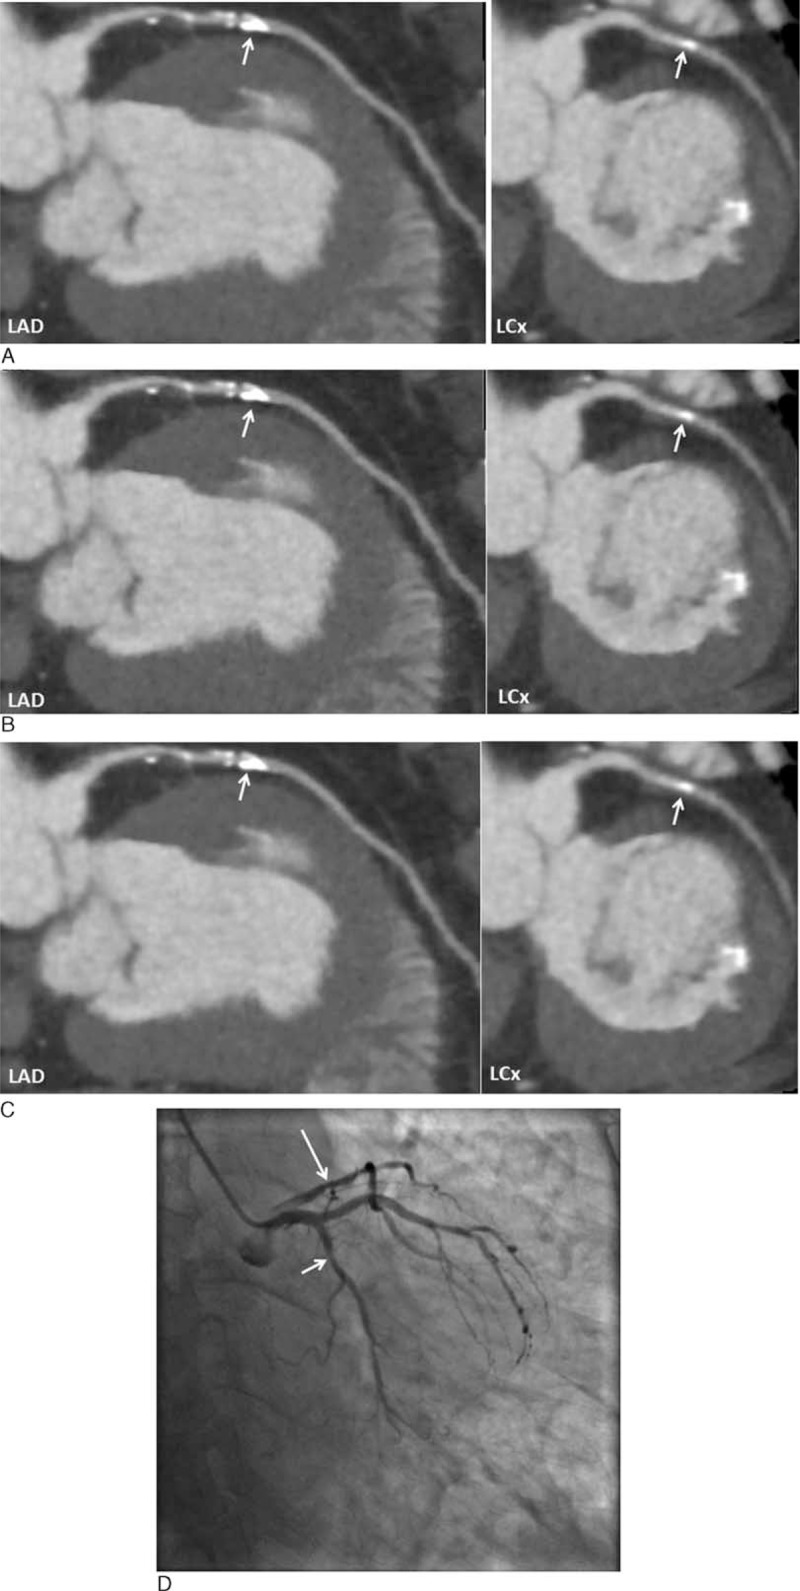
Curved planar reformatted coronary CT angiography (CCTA) shows multiple calcified plaques at the left anterior descending coronary artery (LAD) and left circumflex coronary artery (LCx) in a 75-year-old man. Significant stenosis due to severe calcification was noticed at LAD and LCx with 88% and 68%, 73% and 70%, and 87% and 74% stenoses, corresponding to CCTA-O, CCTA-S, and CCTA_OS, respectively (A–C). Invasive coronary angiography confirms 28% and 45% stenoses at LAD and LCx (D). Long and short arrows refer to the stenosis at LAD and LCx, respectively.
